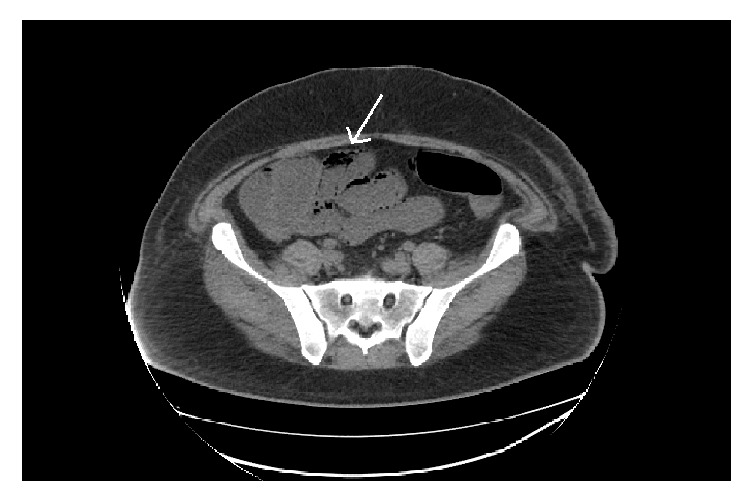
Axial view from CT abdomen exhibiting prominent ascending and transverse colitis with pneumatosis (arrow) of the distal small bowel, perforated abdominal viscus with moderate pneumoperitoneum and pneumomediastinum.
